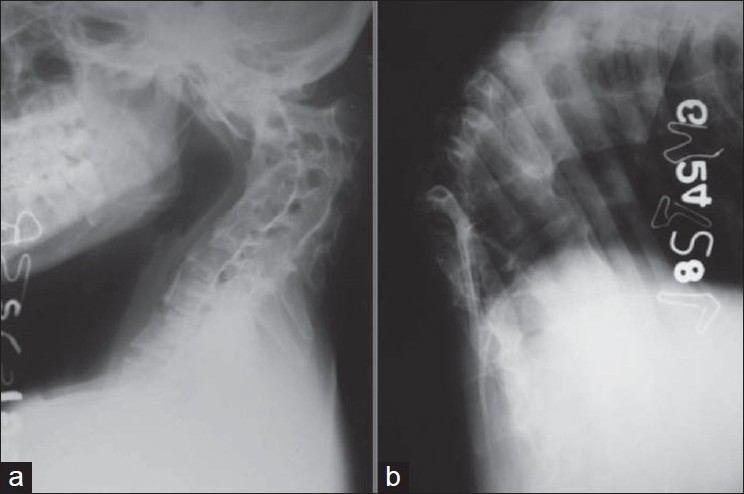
Plain X-ray (lateral view) of cervical spine (a) and dorsal spine (b) shows a severe healed kyphosis of upper cervical spine and dorsolumbar spine as a sequelae of TB spine
